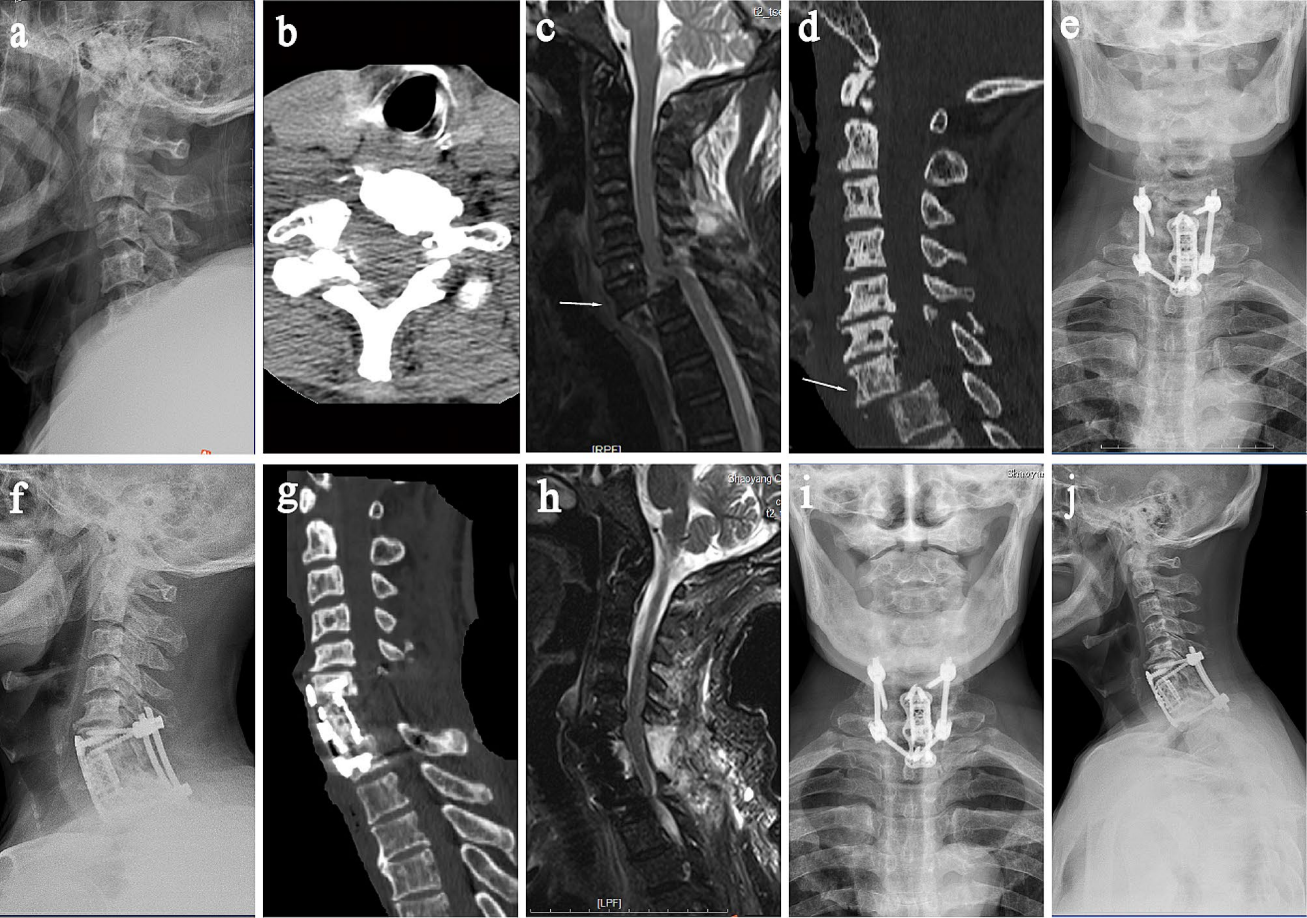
A 73-year-old male patient was admitted to the hospital with “neck pain caused by trauma for 5 hours”. (a) Preoperative cervical lateral X-rays showed no significant abnormalities; (b) Preoperative cervical CT showed that the C7 vertebral body had four degrees of anterior spondylolisthesis and C7 had bilateral adnexa and spinous process fractures; (c) Preoperative MRI plain scan of the cervical spine showed that the C7 vertebral body had four degrees of anterior spondylolisthesis and the cervical pulp at the corresponding level was “S” type, the C6 and C7 accessories were fractured, and the soft tissues of the neck and back were edema; (d) Cervical spine CT examination after traction treatment showed a slight reduction of anterior dislocation of C7; (e, f.) Postoperative cervical X-ray examination indicated that C6 to T1 vertebrae were in a good fixed position, and C7 vertebrae were obviously corrected for forward spondylolisthesis; (g.) Cervical CT examination after surgery indicated that the position of titanium cage and bone grafting were good; (h.) Postoperative cervical MRI showed continuity of spinal cord without obvious compression; (i, j.) Cervical X-ray reexamination 3 months after surgery showed the presence of physiological lordosis of the cervical spine and the position of internal fixation was good
